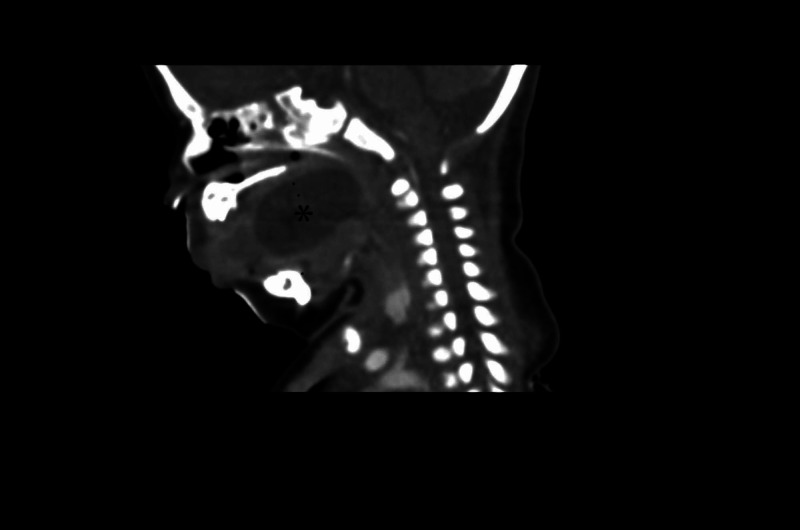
Sagittal CT scan of the neck with contrast demonstrating the hypodense cystic lesion at the posterior aspect of oropharynx (asterisk)
